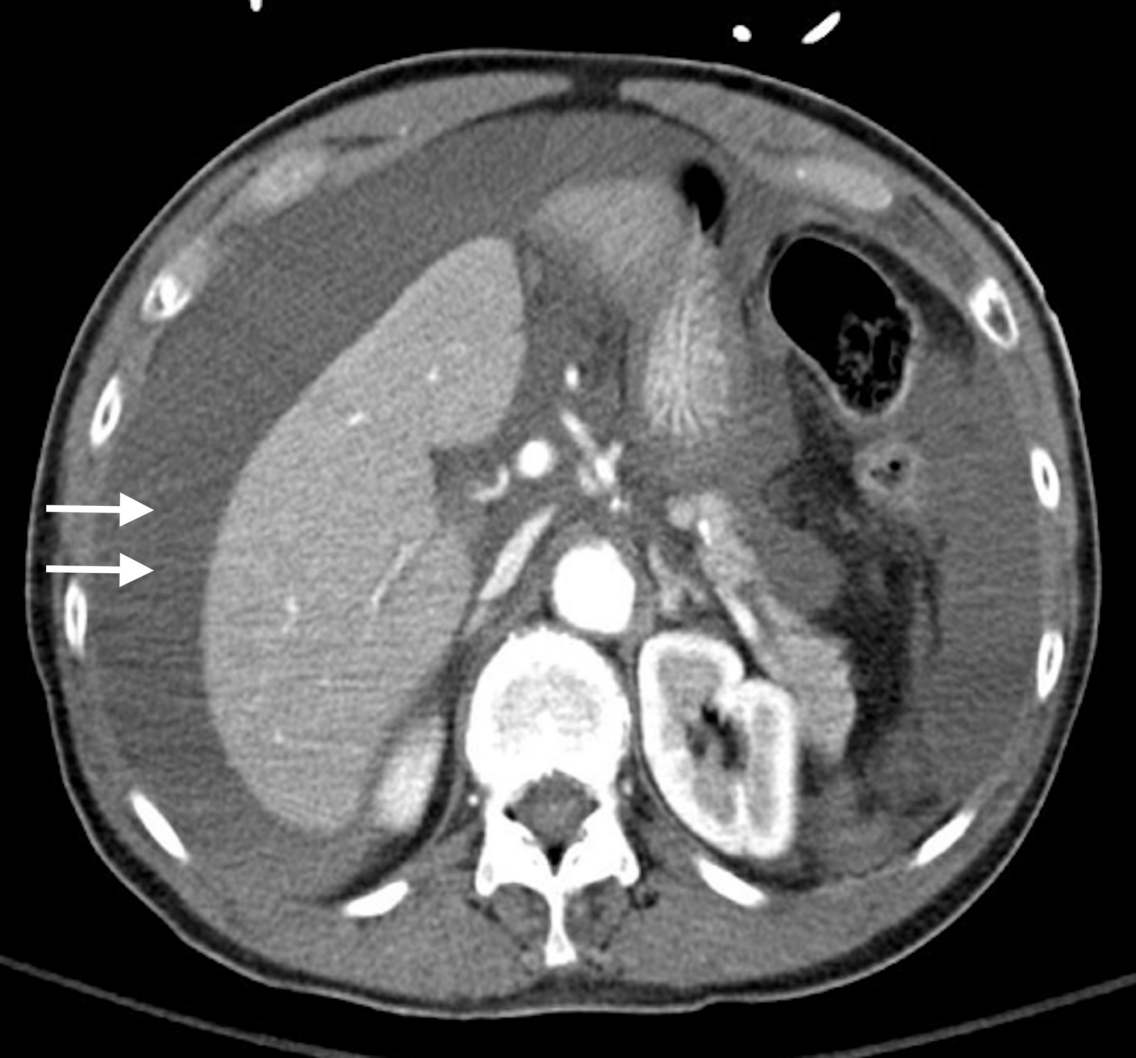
Computed tomography of the abdomen and pelvis. Axial view showing moderate to large amount of low attenuation fluid in the peritoneum, consistent with hemoperitoneum.
